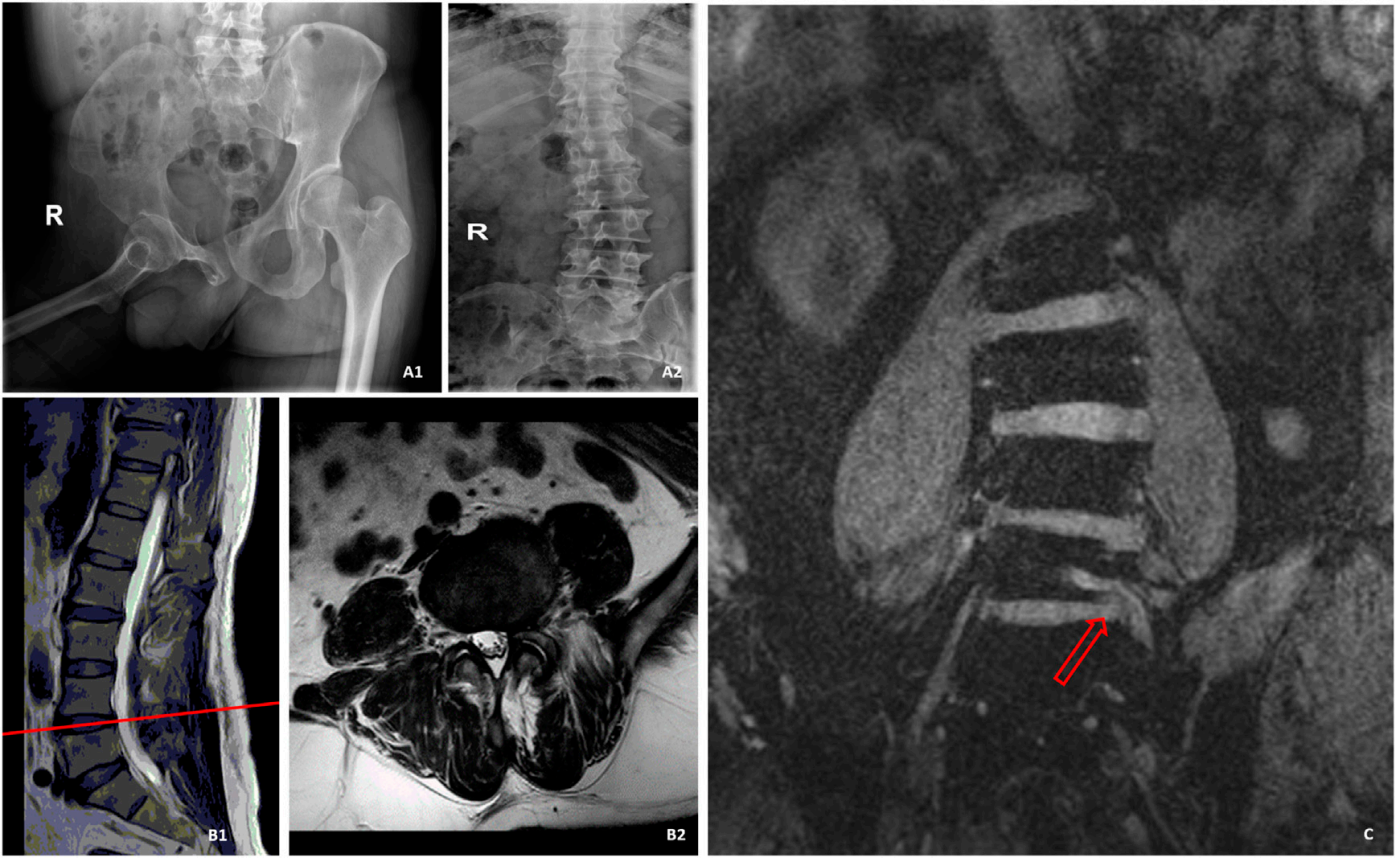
A patient with spinal deformity caused by poliomyelitis, who was admitted to hospital with LBP and radiation pain of left lower limb. (A1) was the pelvic X-ray of the patient. (A2) was the spine radiograph. B was the MR image of the L4/L5 segment of the spine, and no suspicious disc herniation was found. (C) was a 3D-DESS image. Owing to scoliosis, the left L5 nerve root could only be displayed on a single slice, and it could be observed that the left side of L5 nerve root was compressed by a huge nucleus pulposus.
